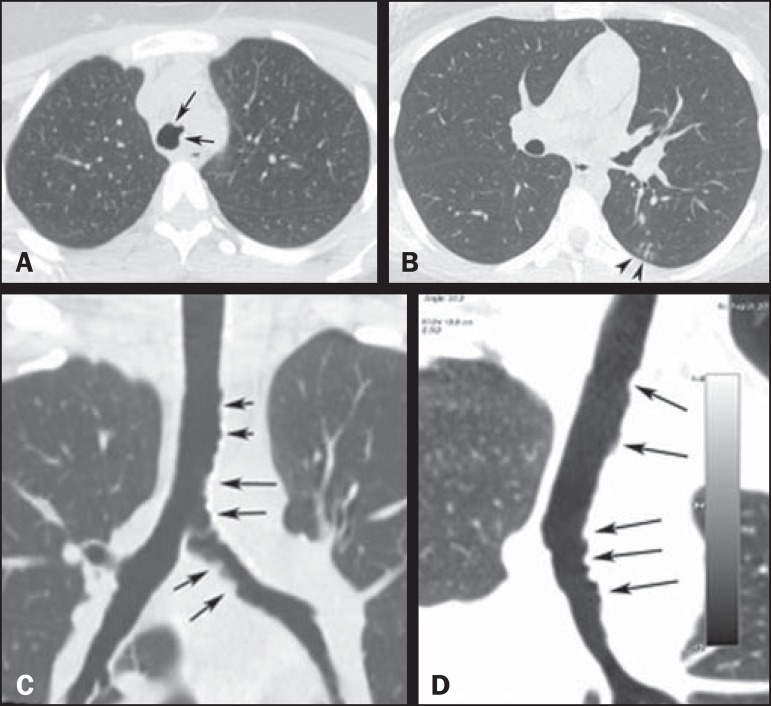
A: Axial CT slice showing irregular narrowing of the tracheal
